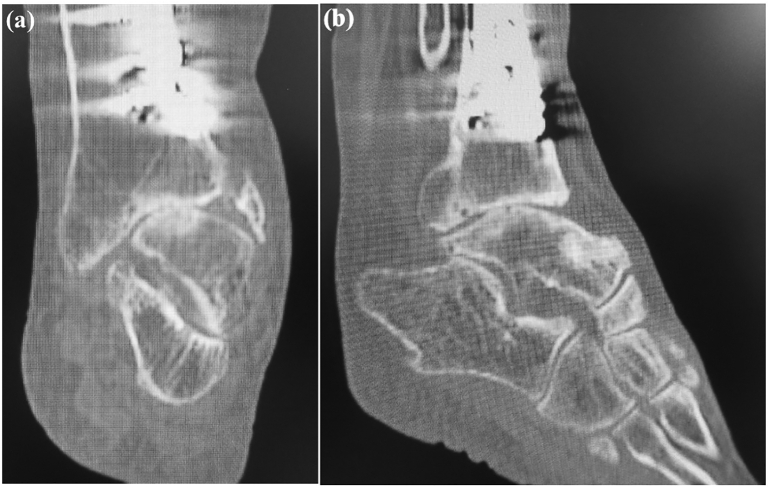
Varus lateral shift of the talus and severe osteoarthritic changes in the left ankle as revealed by (a) a coronal plain computed tomography scan. Severe ankle osteoarthritis, including sclerotic changes and cysts, as revealed by (b) a sagittal plain computed tomography scan.
